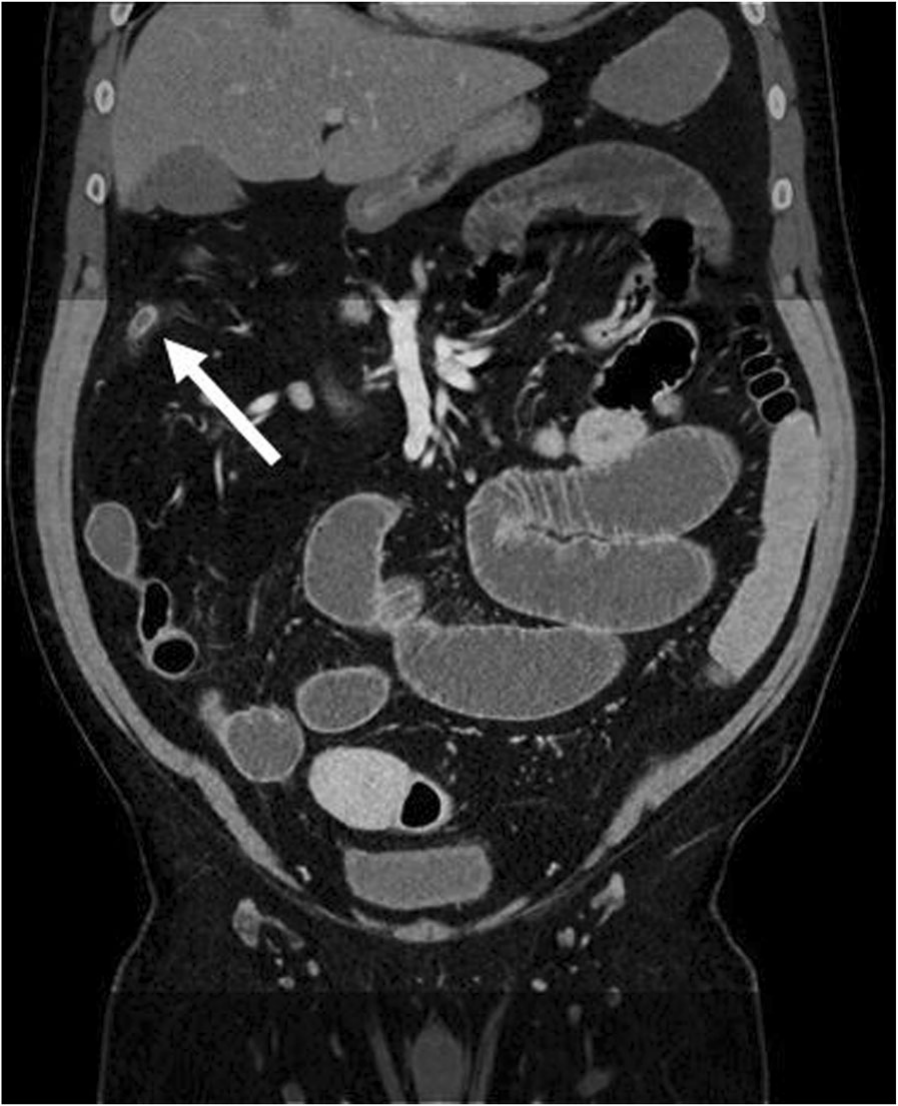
Coronal reformatted CT of a patient with acutely inflamed unusual high positioned appendix as an example for another marginally displayed finding in reduced range CT
